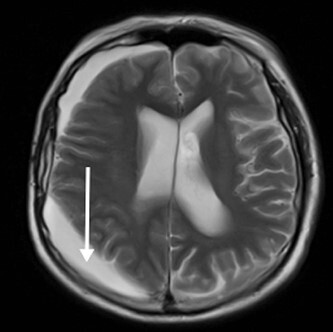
T2W MRI (axial) conducted at 36 months from original presentation demonstrating a right sided subdural collection consistent radiologically to a hygroma (arrow).
